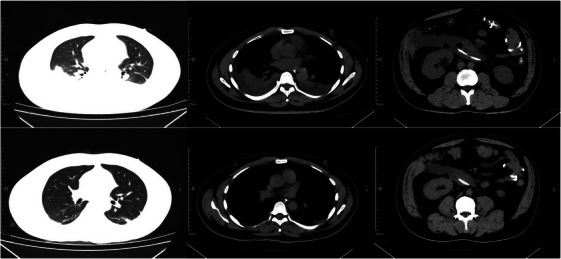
Systemic computed tomography (CT) imaging changes in severe acute pancreatitis (SAP). Imaging changes before and after treatment in a 37‐year‐old male patient with SAP (biliary tract stones, hyperlipidemia).
